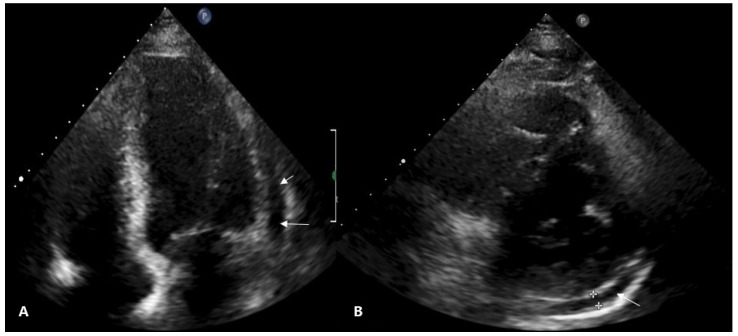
Transthoracic echocardiograms. (A) Four chamber view showing small pericardial effusion (5 mm) adjacent to the lateral wall of the left ventricle (arrows). (B) 5 mm of pericardial fluid along the inferolateral wall (arrow) in parasternal short axis view.
